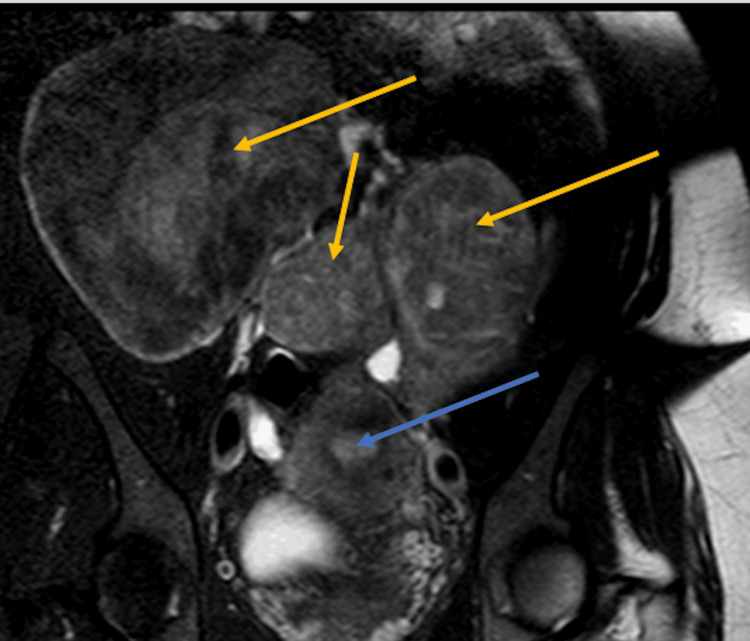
Coronal MRI of the lower abdomen. Blue Arrow: Points to the endometrium of the uterus; Yellow Arrows: Point to the inta-abdominal masses.
